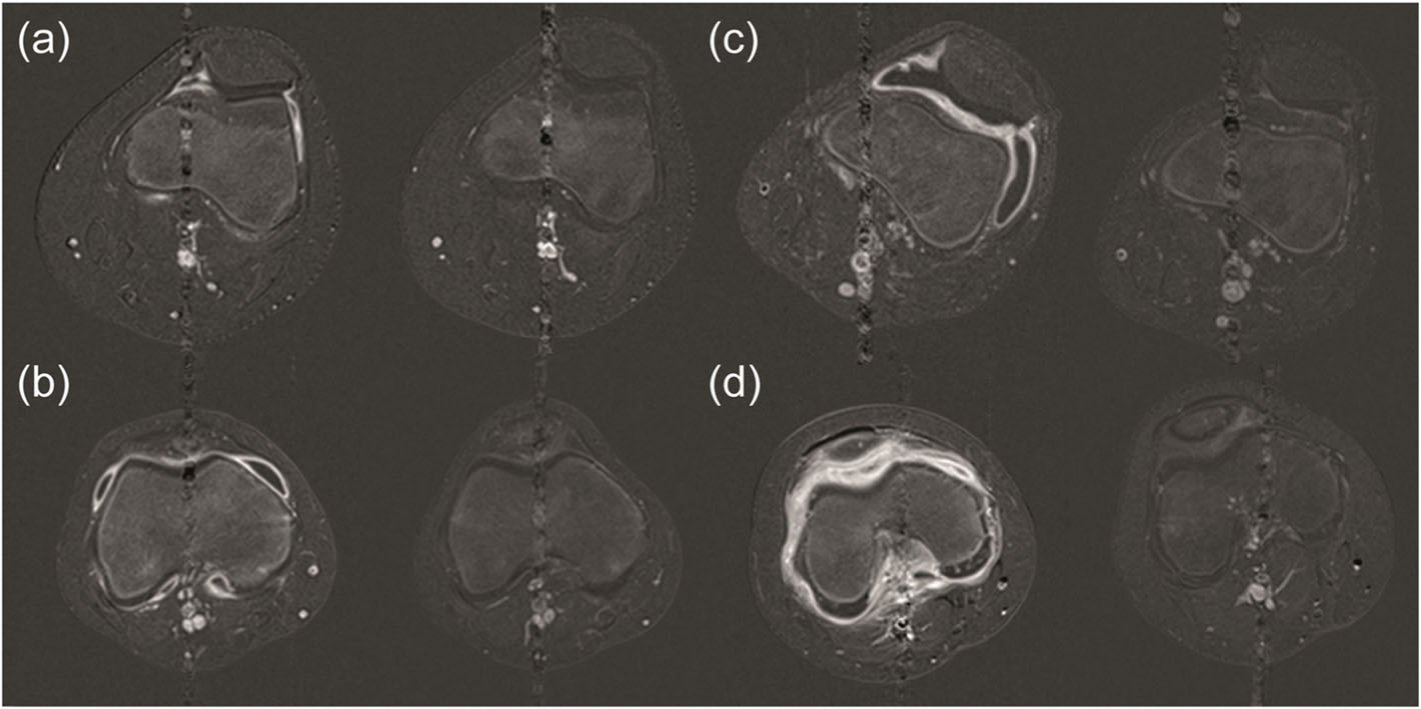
Samples of difference images comparing measured synovial volume before and after IAGI. The difference images were derived from the pre- and post-contrast T1-weighted FS TSE images to highlight the contrast uptake in the synovium for four subjects: (a) 12 year-old female (25.7cm^3^ vs. 0.0cm^3^), (b) 11 year-old male (51.2cm^3^ vs. 0.1cm^3^), (c) 16 year-old female (72.7cm^3^ vs. 0.7cm^3^) and (d) 8 year-old male (101.6cm^3^ vs. 1.9cm^3^). The whole synovial volume is quoted in each case, not just for the slice shown. FS TSE, fat saturated turbo spin echo
